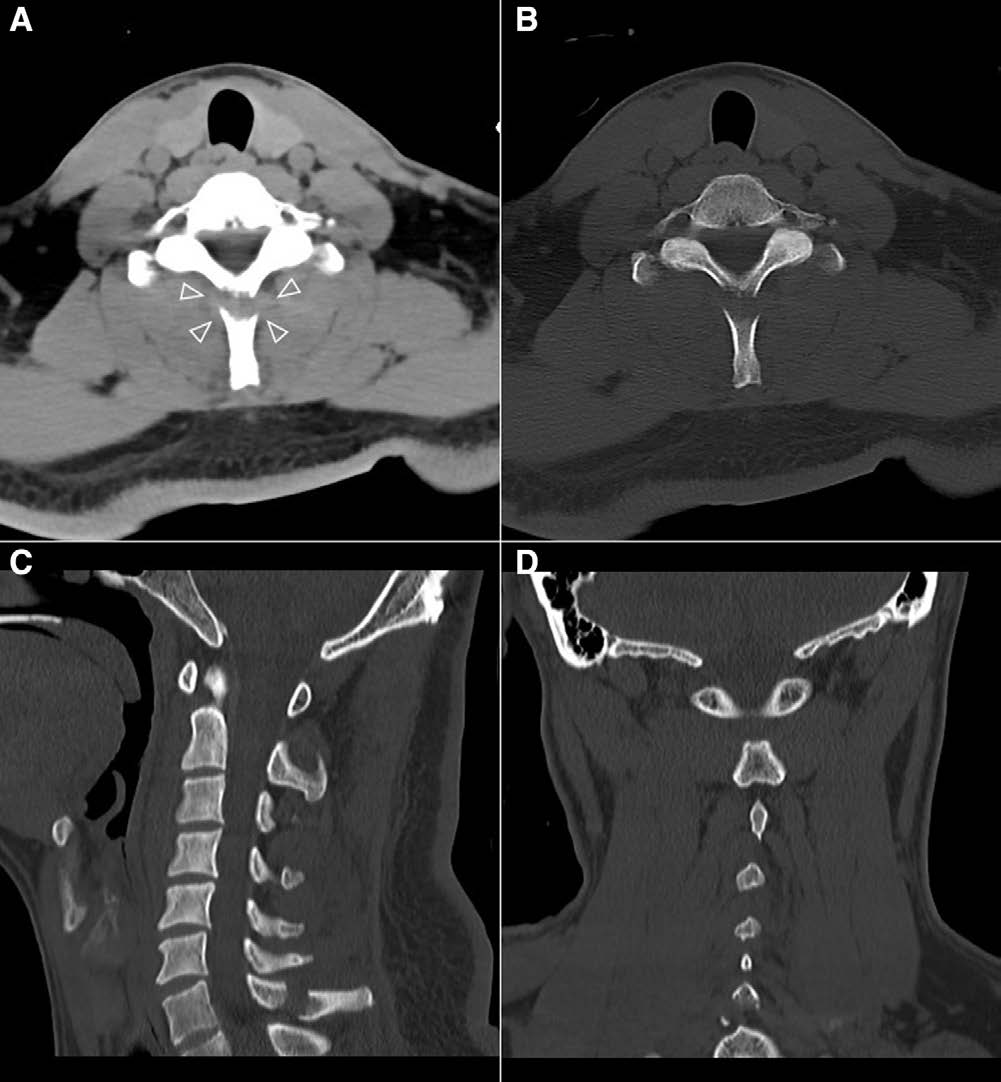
A 35-year-old male alert patient who suffered from a fall injury received a standard cervical CT. The fracture in the C6 spinous process was shown in A (image in soft tissue window on axial plane, WW450/WL300) and B (image in bone window on axial plane, WW1500/WL450). The localized iso-high density shadow at the end of the fracture suggests hematoma formation (△). More detailed spatial anatomical information of the fracture can be better displayed in the MPR(C: sagittal reformatted bone window CT image; D: coronal reformatted bone window CT image, all WW/WL same as B), including the alignment and stability of the fracture. CT = computed tomography.
